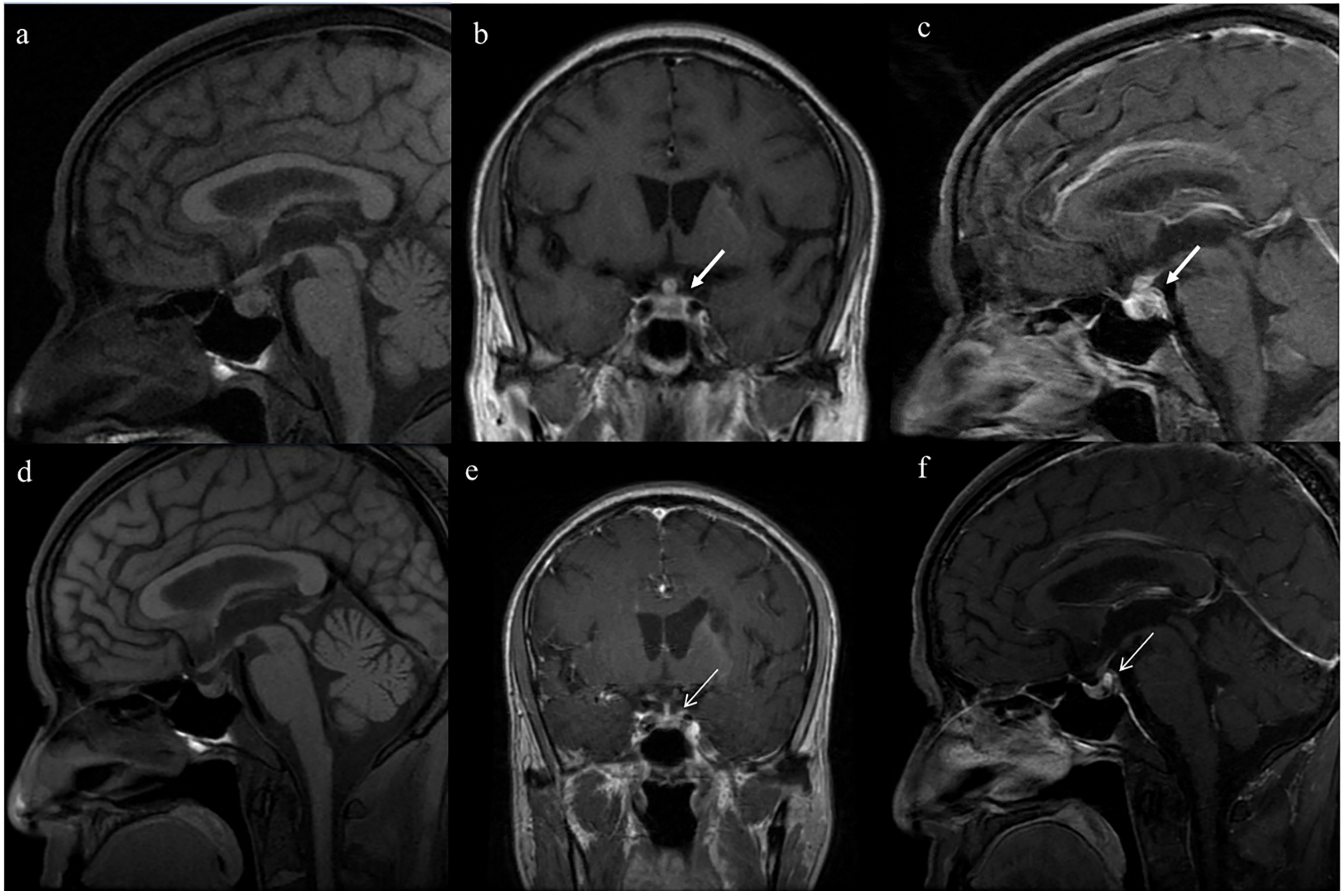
Serial magnetic resonance imaging (MRI) of the pituitary in patient No.9, who fully recovered from diabetes insipidus. Enlargement of the posterior pituitary lobe (5.7mm×7.8mm) and thickening pituitary stalk (transverse diameter 5.3mm) on T1-weighted images (Panels A–C; thick white arrow). After the treatment, the enlargement of the posterior pituitary shrank(3.8mm×4.5mm) and the normalization of the pituitary stalk (transverse diameter 2.3mm) (Panels D–F thin white arrow).
